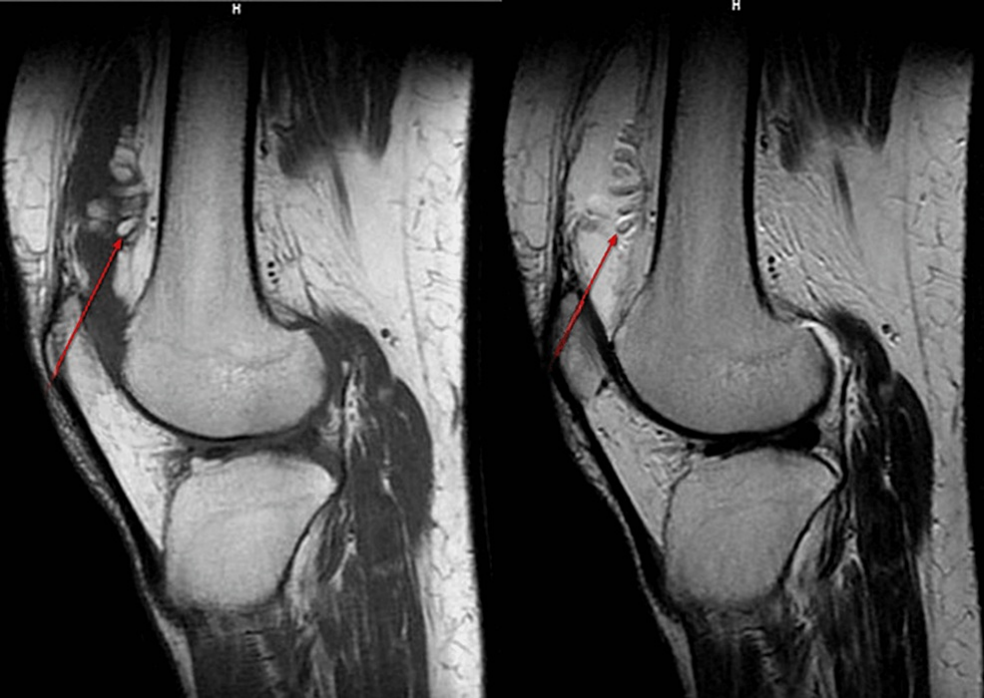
Sagittal T1WI (left) and T2WI (right) MRI showing frond-like projections (red arrow) in the pre-femoral region appearing hyperintense on both sequences. T1WI: T1-weighted image, T2WI: T2-weighted image, MRI: magnetic resonance imaging
